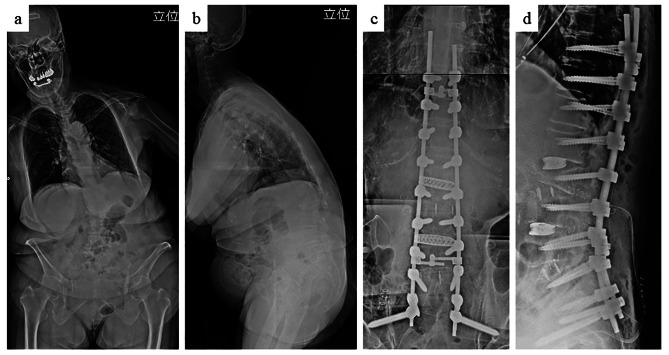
Standing X-ray before (a, b) and during (c, d) the operation
